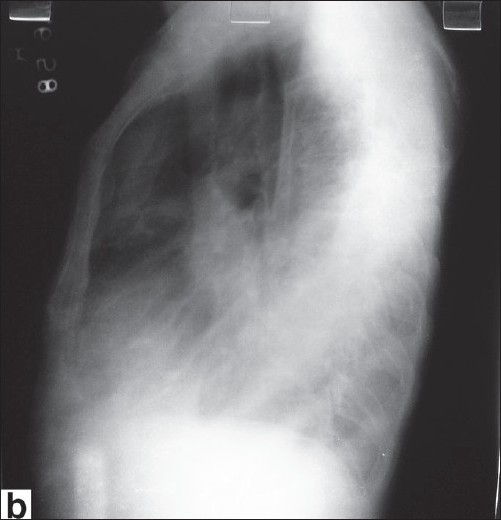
Radiograph chest left lateral view
